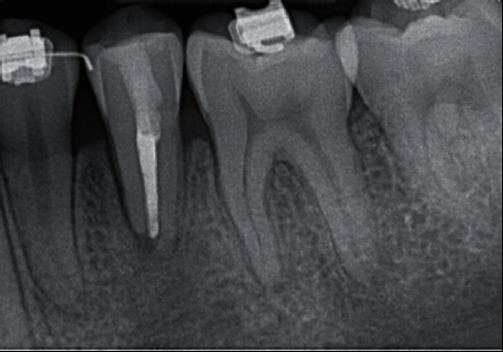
Periapical radiograph of immediate post-operative obturation of tooth #20.
